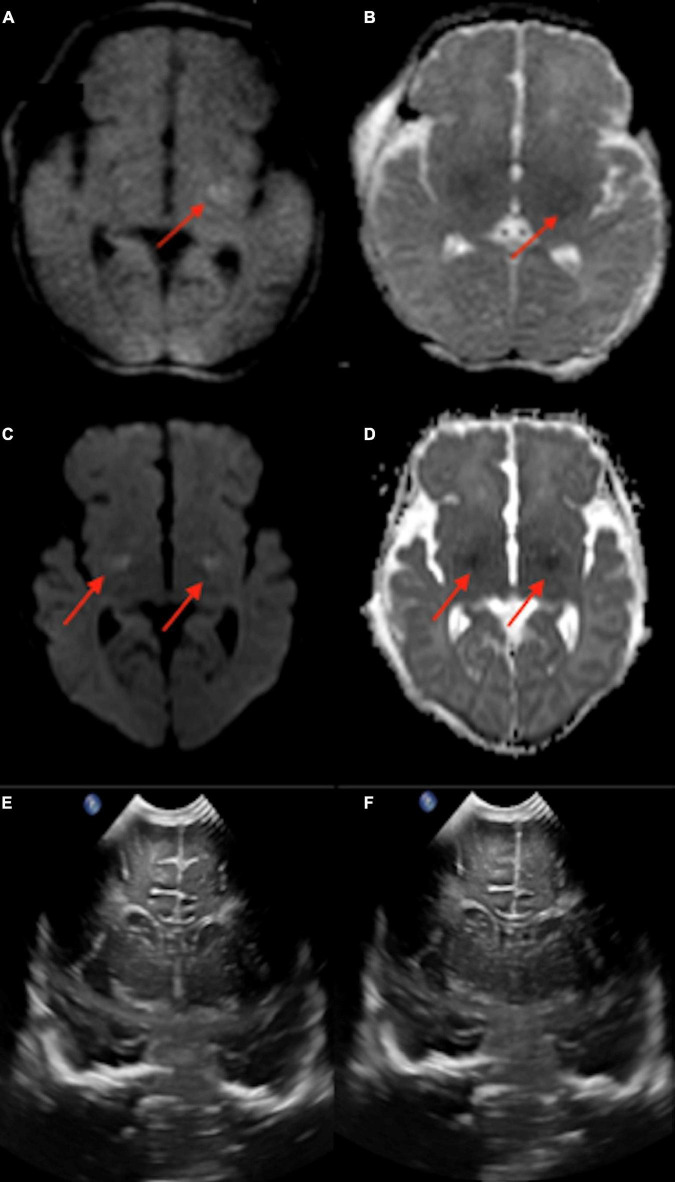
In a 4-day-old term neonate with challenging home birth, followed by transfer to hospital, resuscitation, and therapeutic hypothermia, the point-of-care 1-Tesla DWI (A) and ADC (B) showed reduced diffusion in the left basal ganglia. The next day (5 days old) follow-up 3-Tesla DWI (C) and ADC (D) showed ischemic changes and reduced diffusion in bilateral basal ganglia. Transcranial ultrasound was normal on the first day after birth (E,F).
